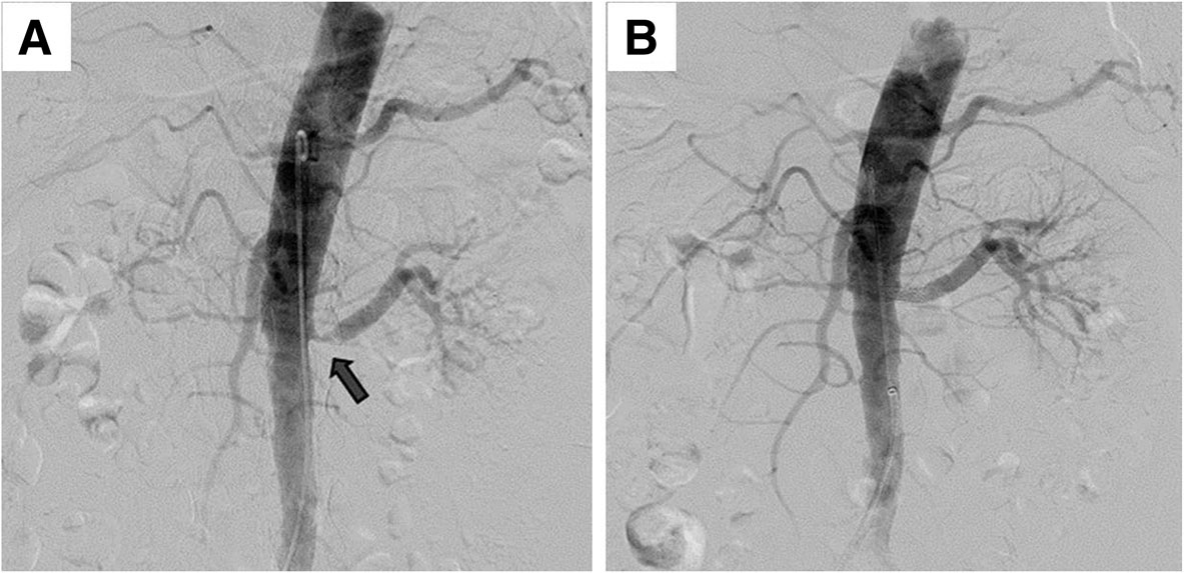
a Renal artery CT angiogram showing severe stenosis of the left renal artery at its site of origin (80%, arrows). b Successful insertion of a stent into the left renal artery
